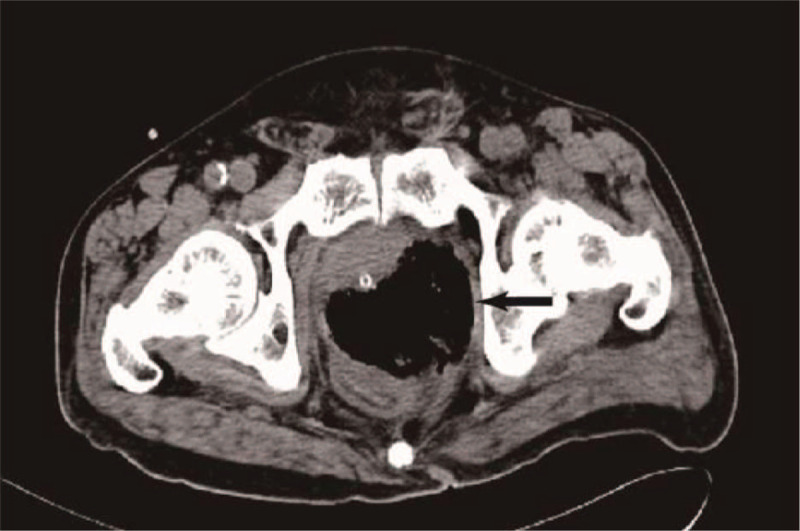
Pelvic CT revealed a progress of gas and purulent exudates collection in the prostate gland (arrow) on day 14 of admission (after aspiration).
